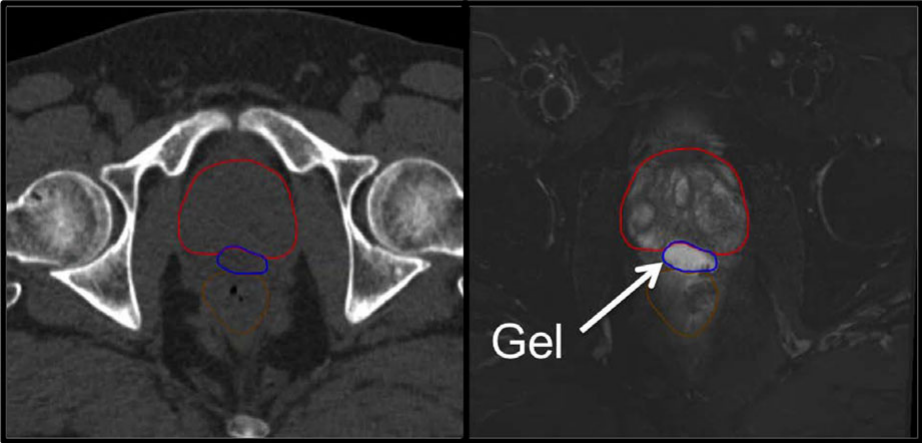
Left: treatment planning CT (TPCT), indicating the prostate (red), hydrogel (blue), and rectum (brown). Right: MRI acquired on the same day to visualize the hydrogel.
